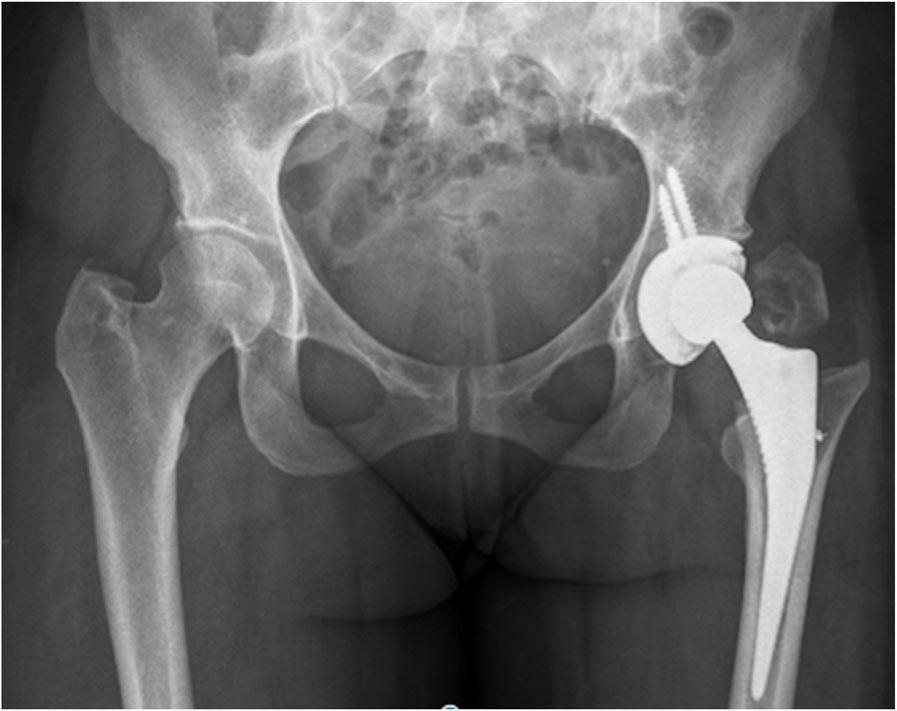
X-ray of postoperative displacement of trochanteric fracture in the case of dysplastic hip
